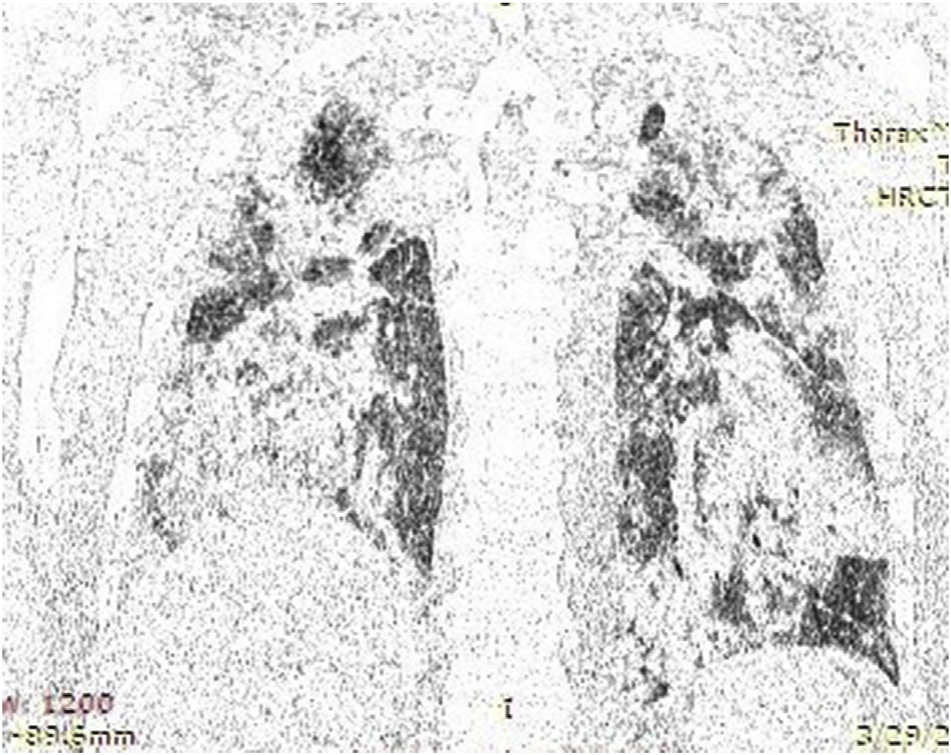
CT-scan (coronal section) showing diffuse ground glass opacity with multiple areas of consolidation.
